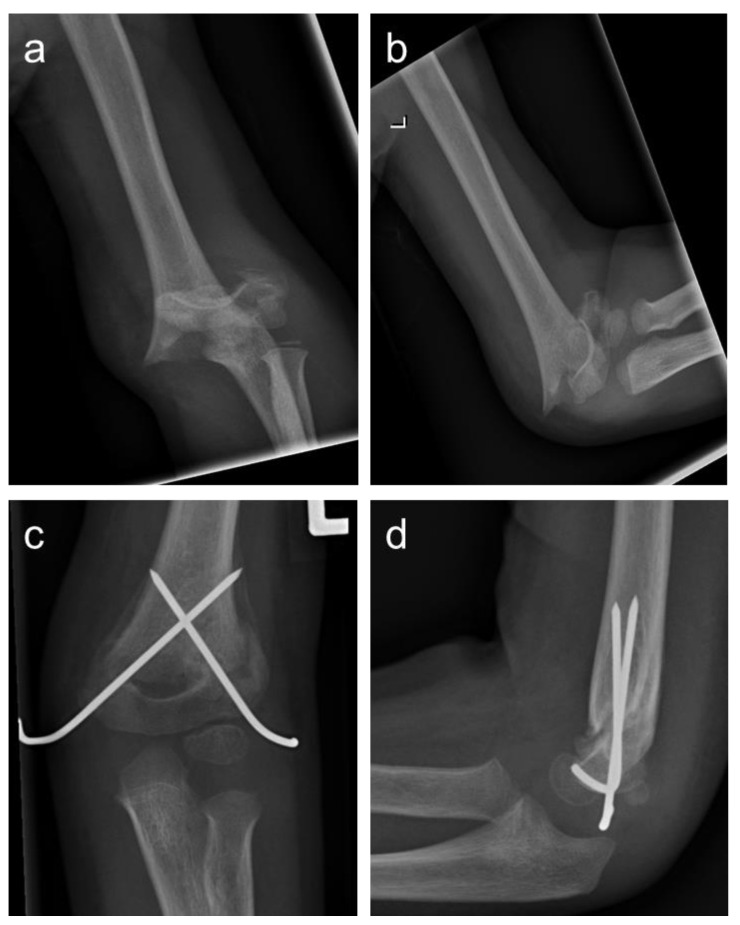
Anterior–posterior and lateral radiographs of a Gartland type 3 supracondylar humeral fracture (a,b); the patient was treated with PCP using 2 Kirschner wires (c,d).
